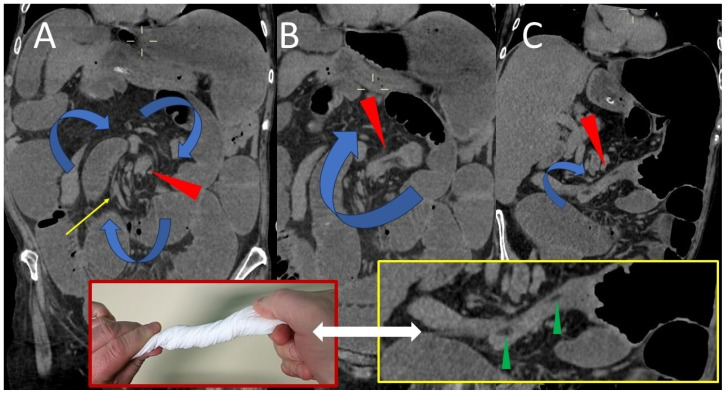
Coronal (A) CT image demonstrates a vortex composed of the cecum entangled on its longitudinal axis and a spiral of mesenteric vessels (yellow arrow) rotating consensually with the cecum: the “whirl” (red arrowhead). Seen along the longitudinal course, the entangled cecum (red arrowheads in (B,C)) contains small hypodense areas between its collapsed and intertwined walls, which represent mesenteric tissue incarcerated by the spiral braid of the cecal walls (green arrowheads in the yellow box).
